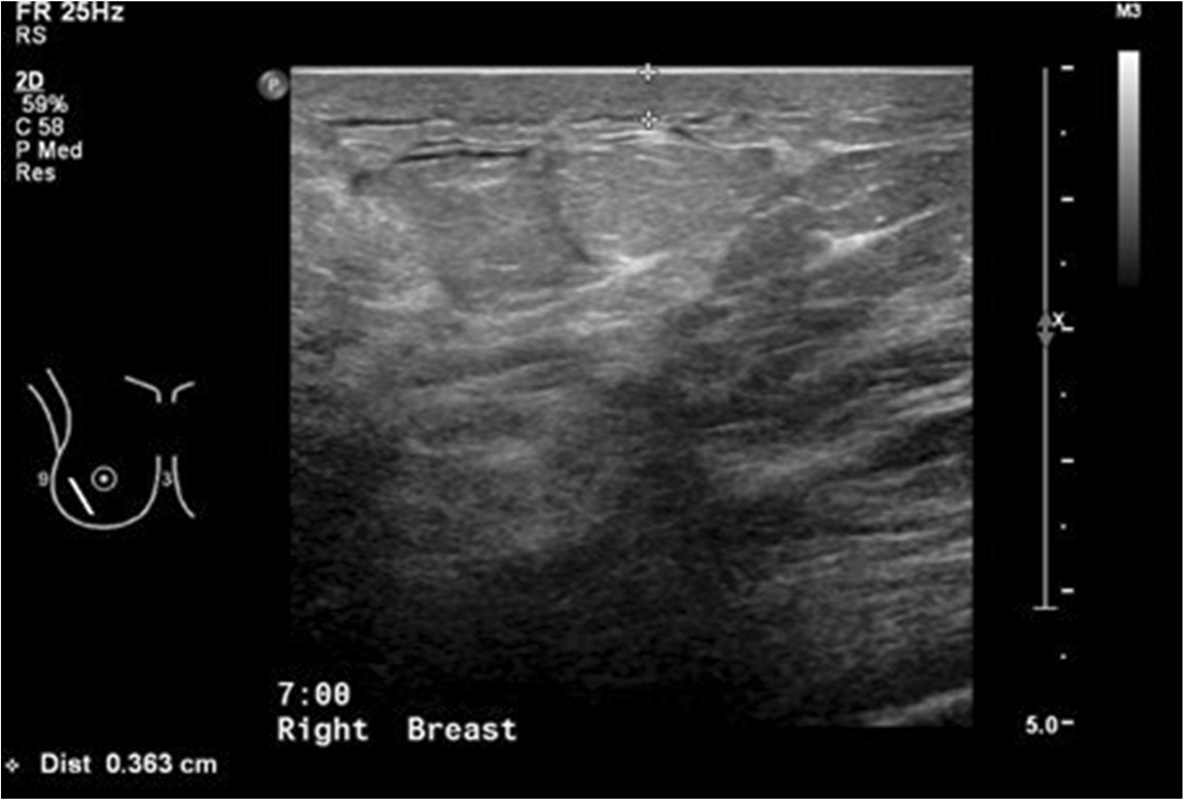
Right breast ultrasound showing skin thickening and underlying marked inflammatory changes with increased echogenicity.
